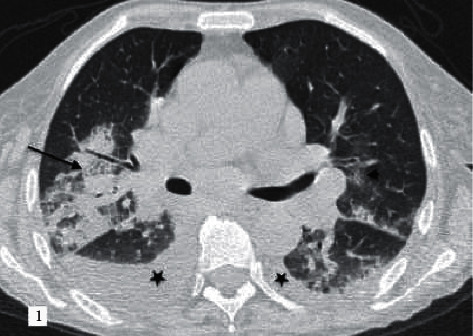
A 70-year-old female patient diagnosed with HCoV-OC43 pneumonia and chronic lymphocytic leukemia (CLL). According to the RSNA guidelines, CORADS score is given as 5. GGO (crazy paving) (black arrow) accompanied by interlobular and intralobular septal thickening on the axial CT section and patchy consolidation areas, faint GGO areas (black arrowhead), and pleural effusion (asterisks).
